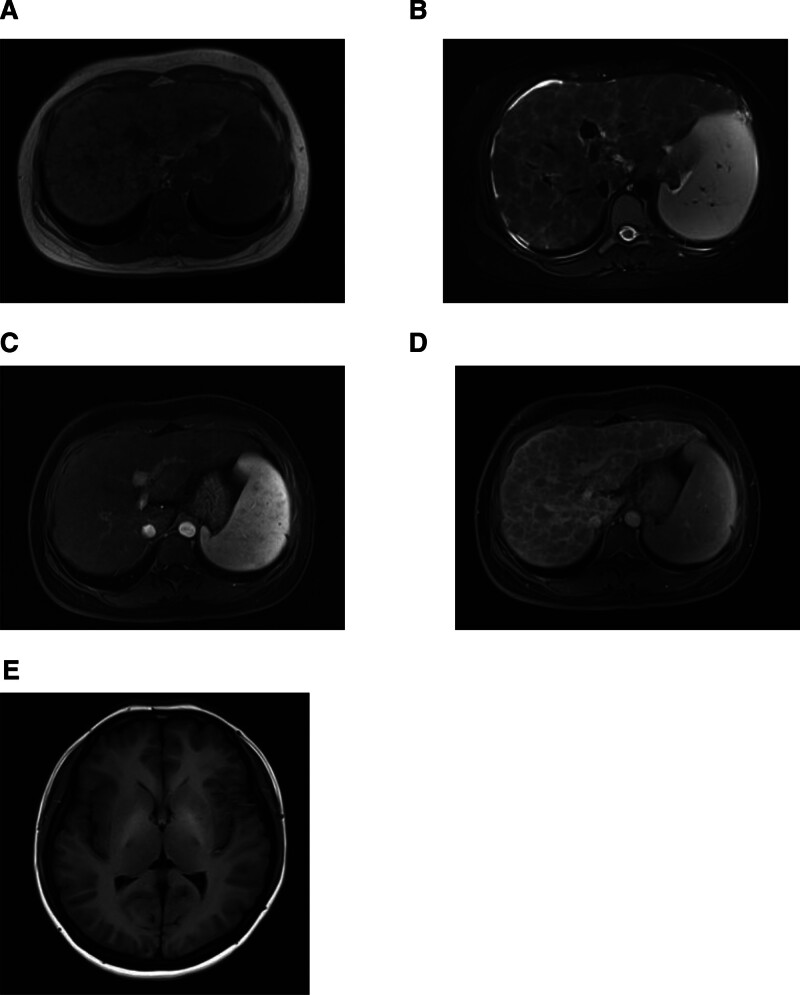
Hepatic and cerebral magnetic resonance imaging. Diffuse nodules appear distributed in the hepatic parenchyma, separated by fibrous septa, with an isointense signal on T1-weighted imaging (A), and a hyperintense signal on T2-weighted imaging (B). The nodules are not enhanced during the arterial phase (C). The fibrous septum is more pronounced in the venous phase (D). Bilateral pallidus abnormalities are observed on T1 head imaging (E).
